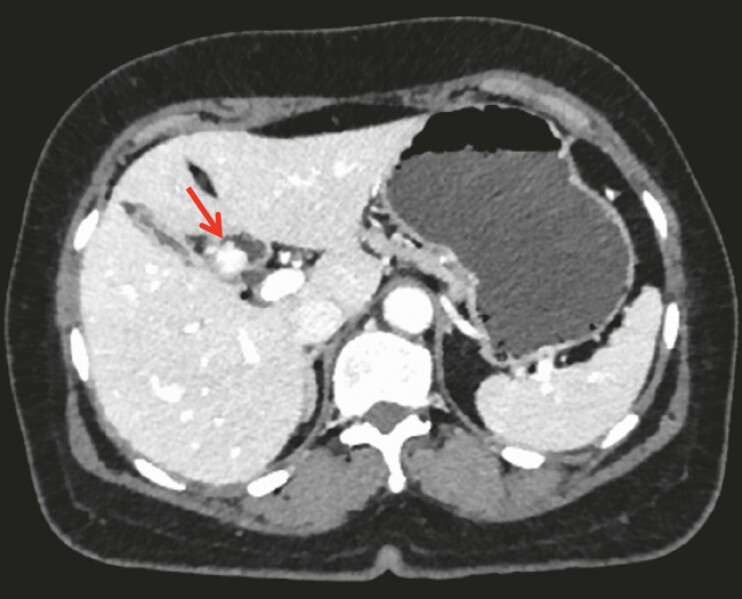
A contrast-enhanced CT image revealing a well-defined, enhancing nodule at the superior aspect of the duodenal bulb (arrow). CT, computed tomography.
